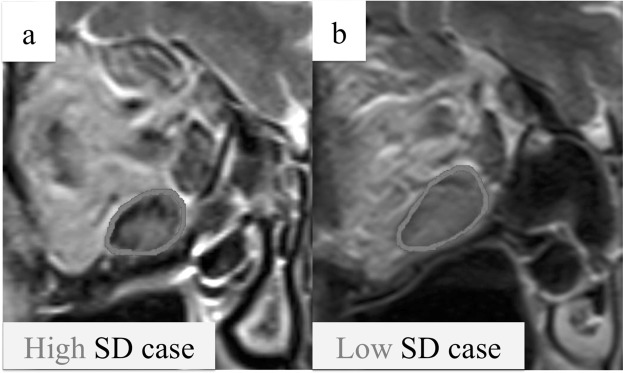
Definition of ROI and representative cases of high and low SD. The ROI was placed along the perimeter of the greatest enlarged extraocular muscle in a coronal section T2WI, and the SD of the inside signal intensity was measured. (a) A representative case of high SD. (b) A representative case of low SD. ROI, region of interest; SD, standard deviation; T2WI, T2-weighted image.
